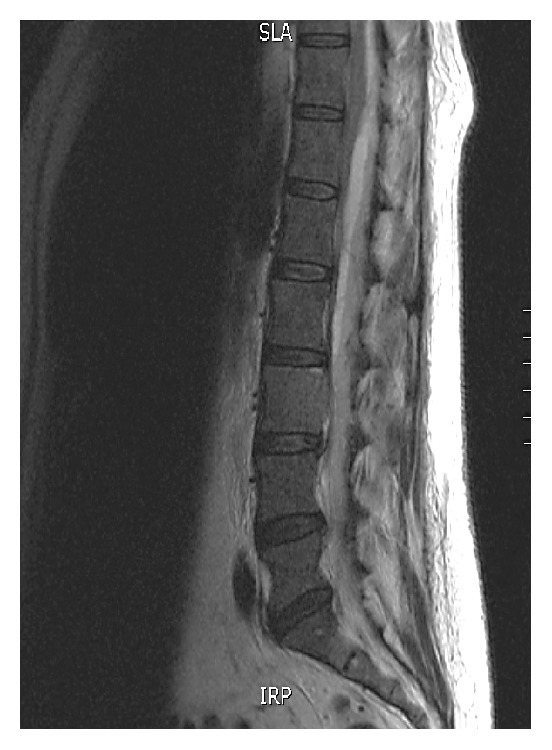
MRI of the lumbar spine in sagittal projection showing critical long segment spinal cord compression extending approximately from the T12, left paraspinal intramuscular hematoma level, inferiorly to the L5.
